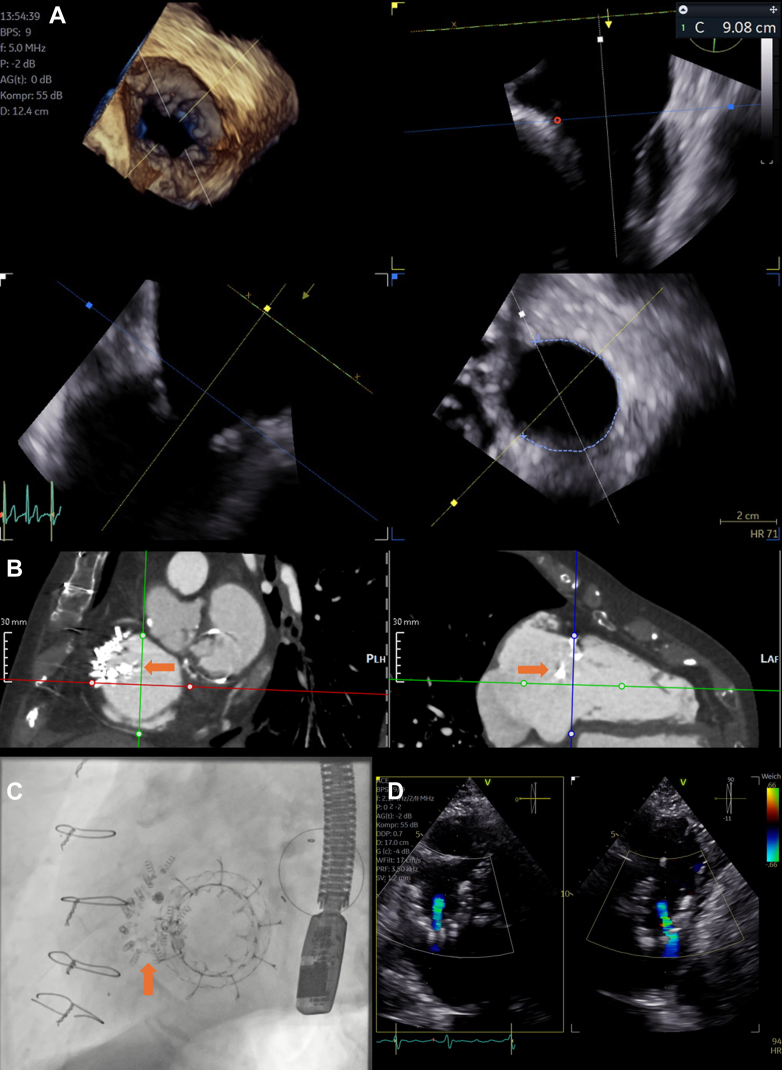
Pre-, Peri- and Postinterventional Imaging for Patient 1 (A) Three-dimensional transesophageal echocardiography demonstrates annuloplasty coverage measurement of the tricuspid annulus, starting anteriorly, as close to the aorta as possible, right to the opening of the coronary sinus. (B) Cardiac computed tomography angiography short-axis and long-axis views demonstrate dislocation of the annuloplasty device (orange arrows). (C) Fluoroscopic view at the end of the procedure with the dislocated annuloplasty device (orange arrow) and orthotopic transcatheter tricuspid valve replacement. (D) Transthoracic echocardiography reveals final result with mild to moderate prosthetic valve regurgitation.
